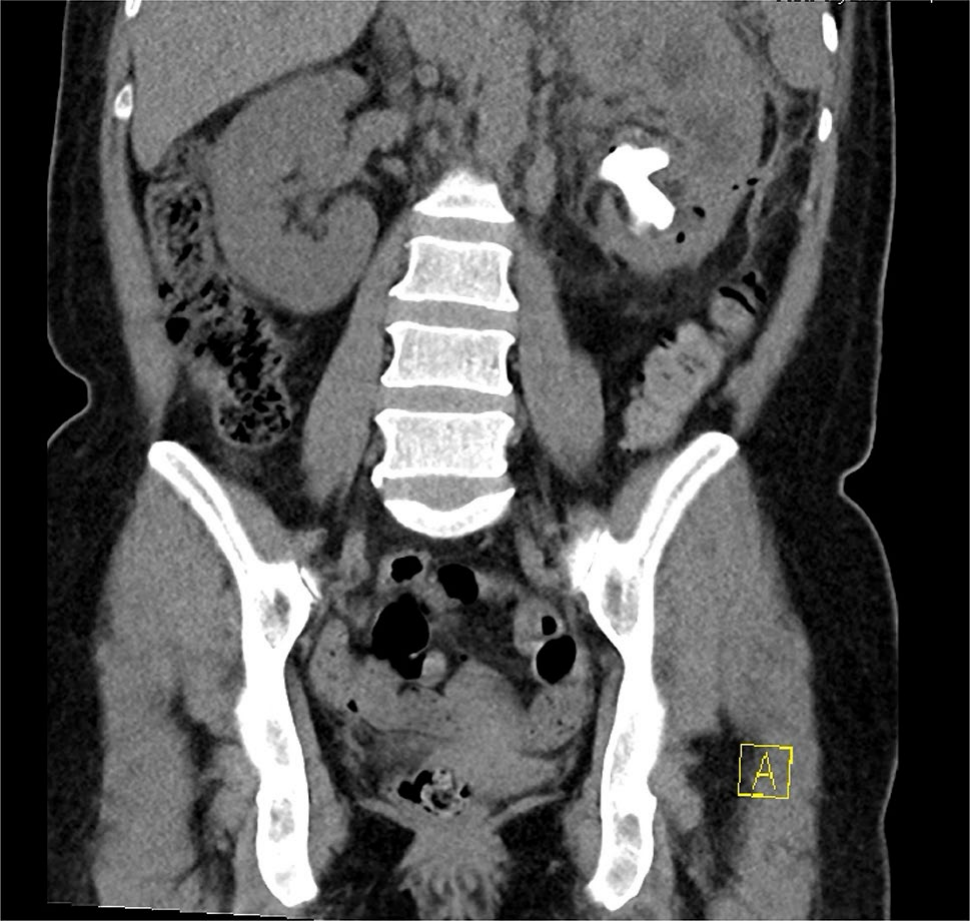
Computed tomography scan of the abdomen and pelvis showing emphysematous pyelonephritis of the left kidney with marked hydronephrosis. Partial staghorn calculus left kidney
